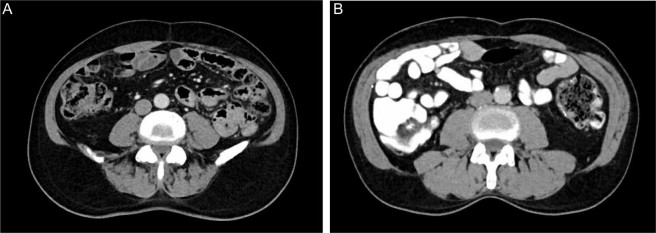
CT images of two patients with either water only or Télébrix Gastro as oral contrast. (A) shows an axial image of an abdominal CT of a 72-year-old man with metastatic renal cell carcinoma where the gastrointestinal tract was filled with water only (grey lumen). (B) shows an axial image of an abdominal CT of a 65-year-old man colorectal liver metastasis where the gastrointestinal tract was filled with Télébrix Gastro (white lumen).
